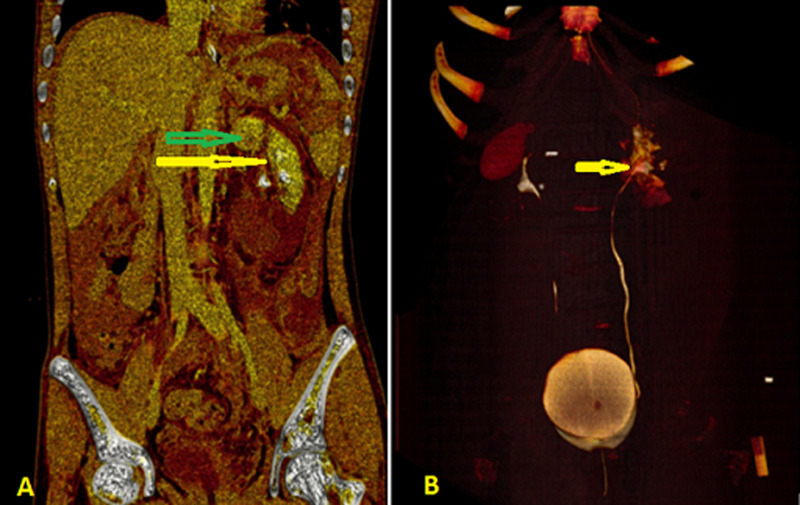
three (3-D) reconstructed computed tomography images showing the extent of injuries (arrows)
